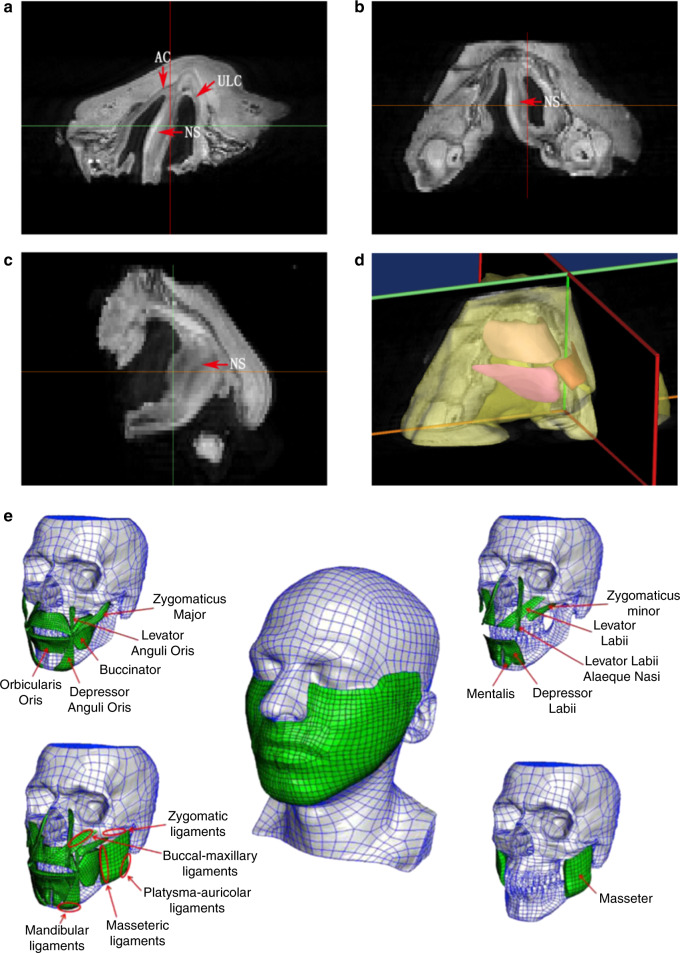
Nasal cartilage and facial muscles obtained from MRI scans. a–c Micro-MRI scan of the nasolabial region of an induced infant with unilateral complete cleft lip.^5,7^ The red arrow indicates the position of the cartilage. AC alar cartilage, ULC upper lateral cartilage, NS nasal septum cartilage. d 3D model reconstruction of the nasolabial region based on micro-MRI, including soft tissue and cartilage. e Facial muscles obtained from MRI^37^
